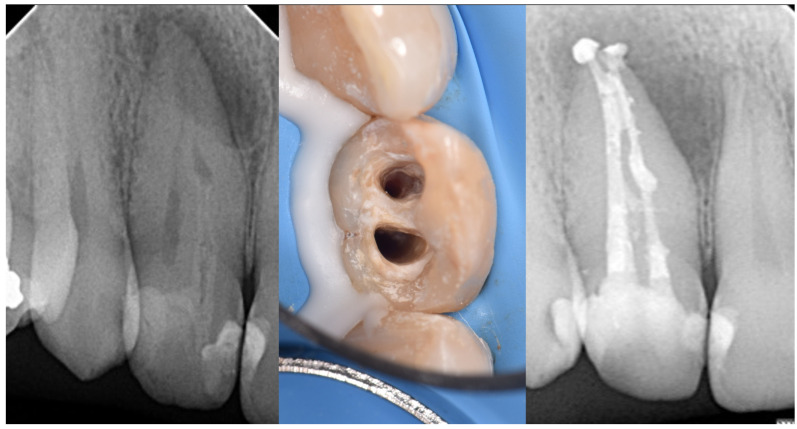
Radiographic images of Oehlers Type IIIb *dens invaginatus* case in maxillary lateral incisor (courtesy of F.C.N.).
